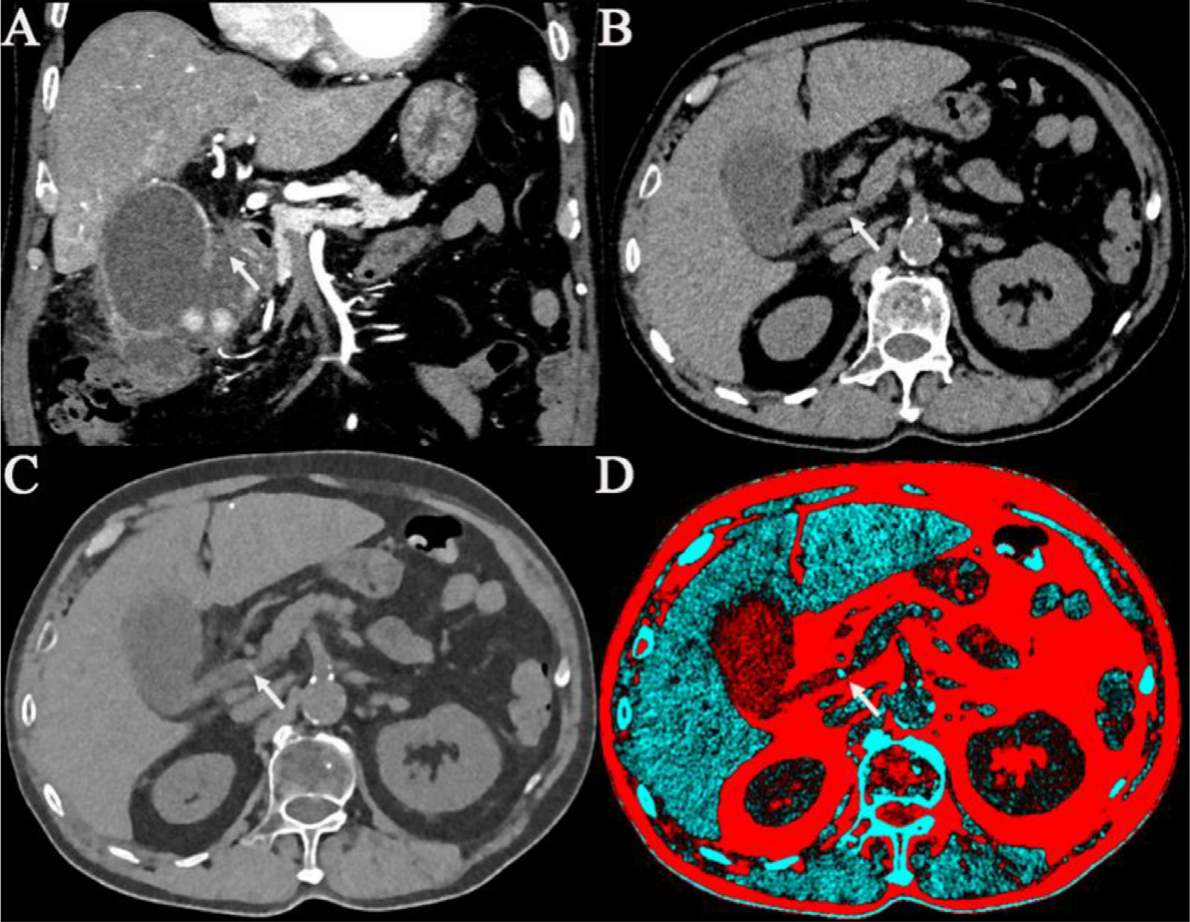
Photon-counting CT (PCCT) imaging of the abdomen shows gallstones, upper common bile duct dilation, and gallbladder perforation. Contrast-enhanced CT images in the coronal plane demonstrate hyperdense nodules, fluid accumulation around the gallbladder and bile duct walls, which appear thickened with enhancement. Specifically, the PCCT imaging revealed a localized defect in the gallbladder wall, accompanied by pericholecystic fluid collection, indicating bile leakage secondary to gallbladder perforation (A). The 60 keV PCCT image (B) reveals dilation of the common bile duct and suspected common bile duct (arrows). The 120 keV PCCT image (C) shows a more clearly defined common bile duct. A dedicated pseudo-color template for gallstones (D) enhances its visualization (arrows).
